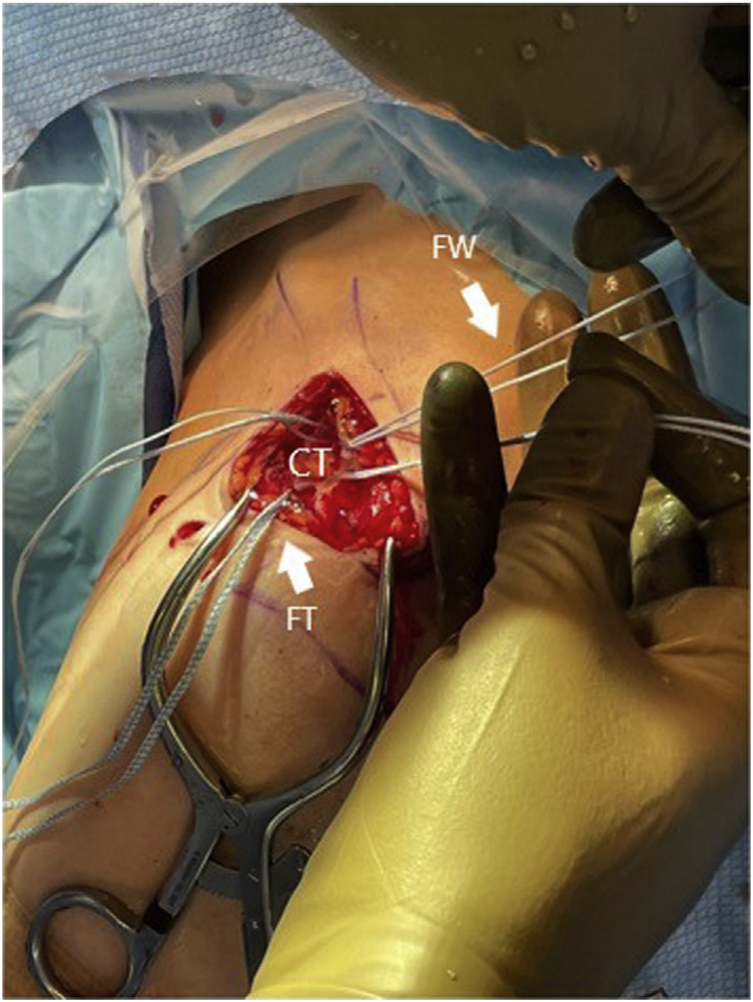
The same left shoulder is shown in the beach-chair position, looking from a lateral perspective. The FiberTape cerclage (FT) and the doubled-over #5 FiberWire (FW) are both pictured after passage around the coracoid (CT). The surgeon can be seen as he passes the tales of the FiberWire through its looped end to create a luggage tag around the coracoid.
